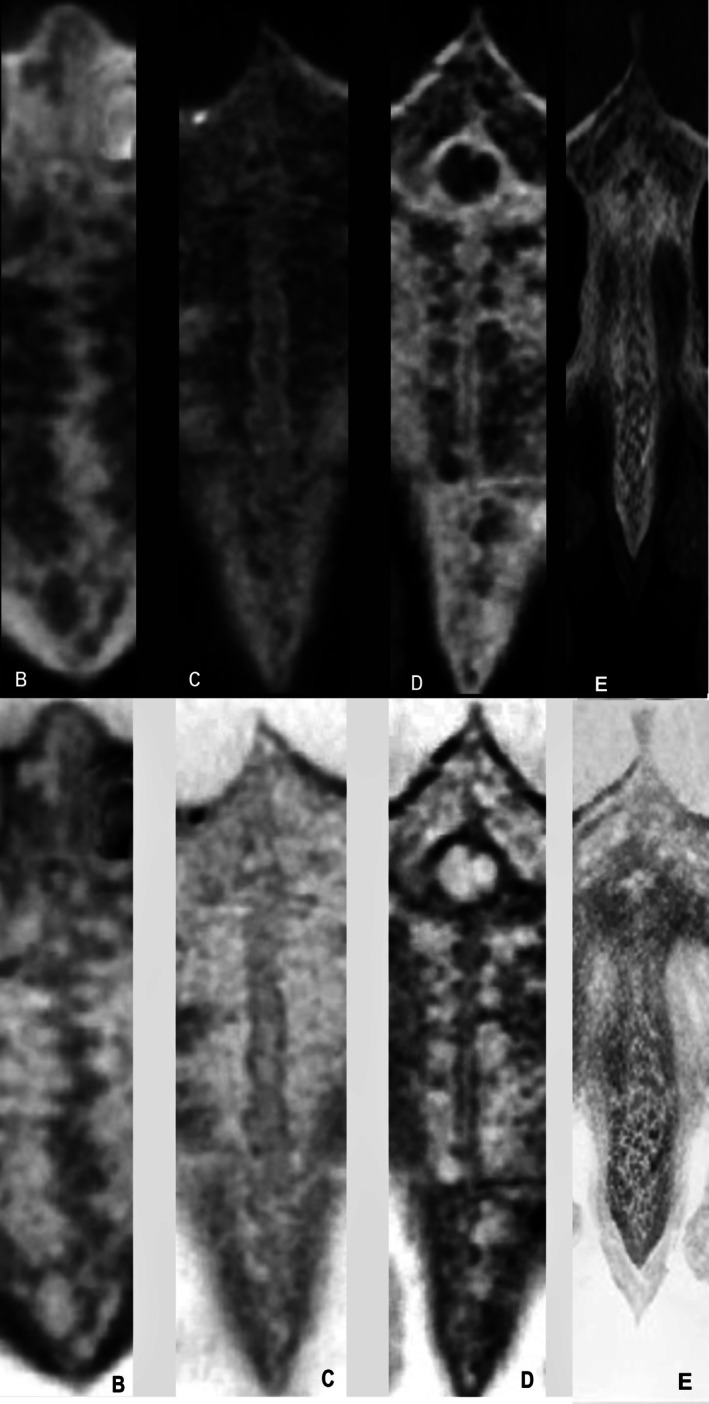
Representative cone‐beam computed tomography (CBCT) images of midpalatal suture maturation stages (B, C, D and E). Top row: Unfiltered images; bottom row: Images displayed with a negative filter to enhance suture boundary visualisation.
